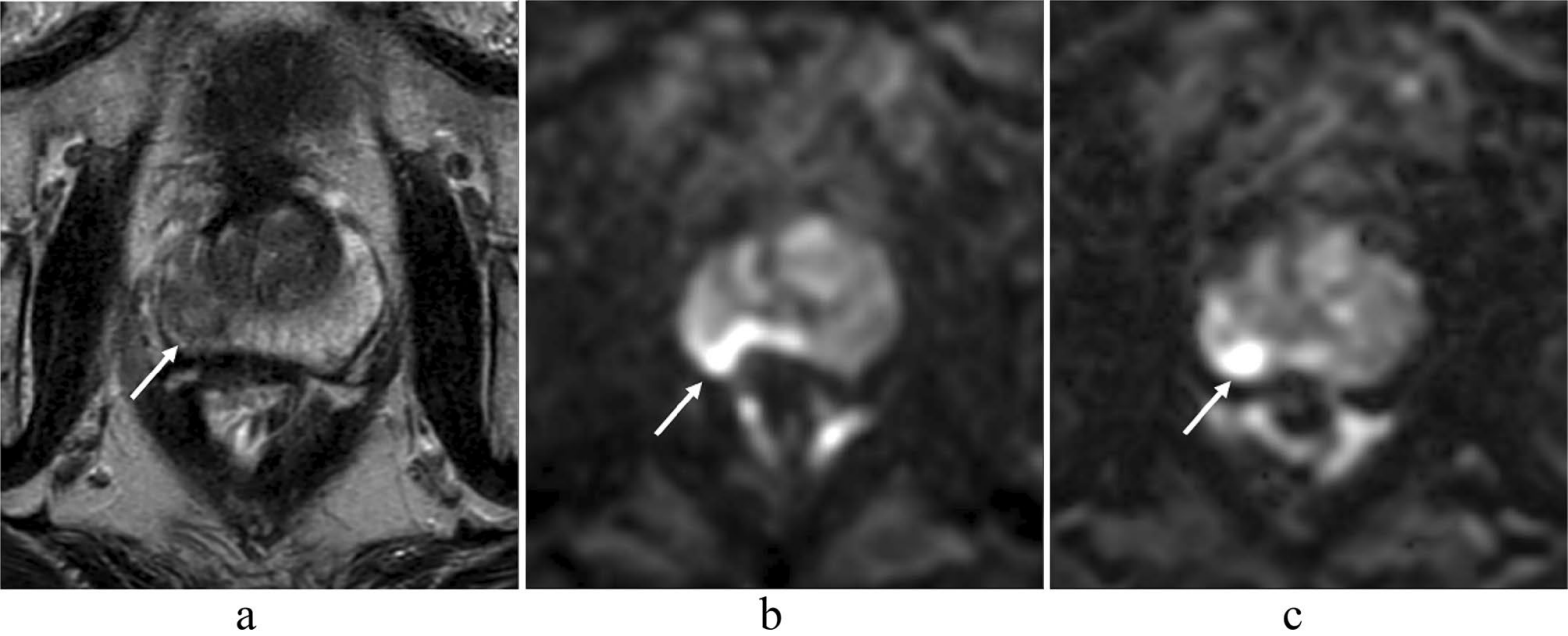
A 73-year-old man with prostate cancer (PSA level, 8.34 ng/mL; Gleason score, 3 + 3) in the right peripheral zone. A heterogeneous hypointense lesion with mass effect is seen on T2-weighted imaging (a) (arrow). A focal hyperintensity is depicted clearly on msEPI DWI (c) (arrow). Lesion clarity in ssEPI DWI (b) is impaired by distortion induced by rectal gas (arrow). However, SNR is higher in ssEPI DWI (b) than in msEPI DWI (c). *PSA* prostate-specific antigen, *SNR* signal-to-noise ratio, *ssEPI* single-shot echo-planar imaging, *DWI* diffusion-weighted imaging, *msEPI* multi-shot echo-planar imaging.
